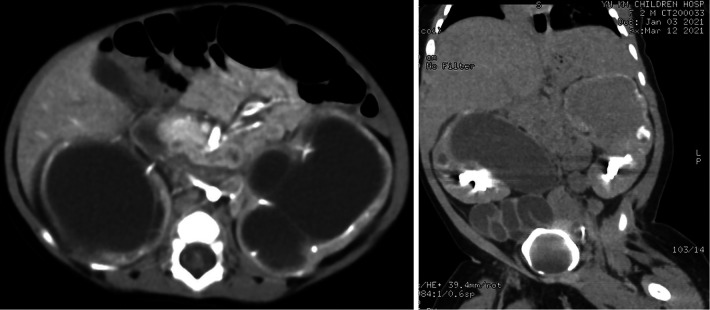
Results of preoperative CT showed severe hydronephrosis and ureterectasis of bilateral upper moiety, left ureteral opening, and the right ureterocele.
